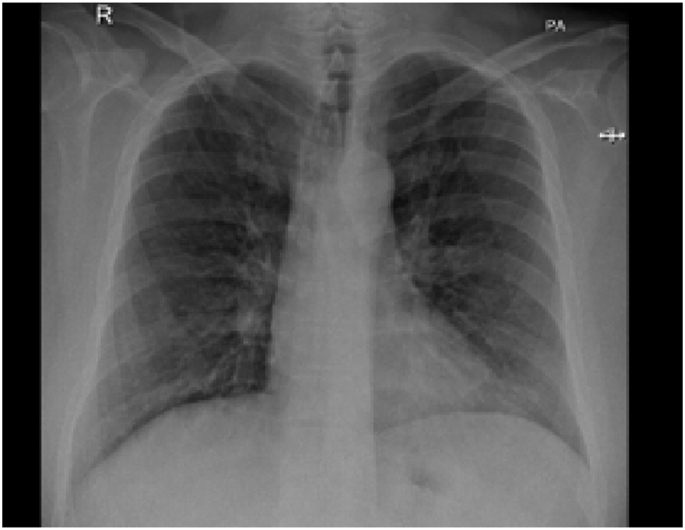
Chest x-ray PA, February 2021: normal.
